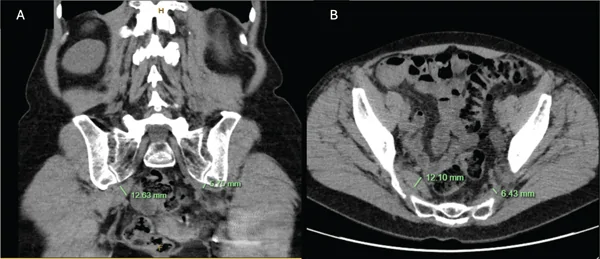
(A,B) Noncontrast CT (A) coronal and (B) axial images demonstrating significant atrophy of the left piriformis when compared with the right. Measurements were taken in both the coronal and axial plane utilising multiplanar reformats and Hounsfield attenuation values to ensure measurements were taken at identical anatomical levels bilaterally. The green markers indicate muscle bulk differential (12.63 vs. 5.75 mm in the coronal plane, 12.10 vs. 6.43 mm in the axial plane).
